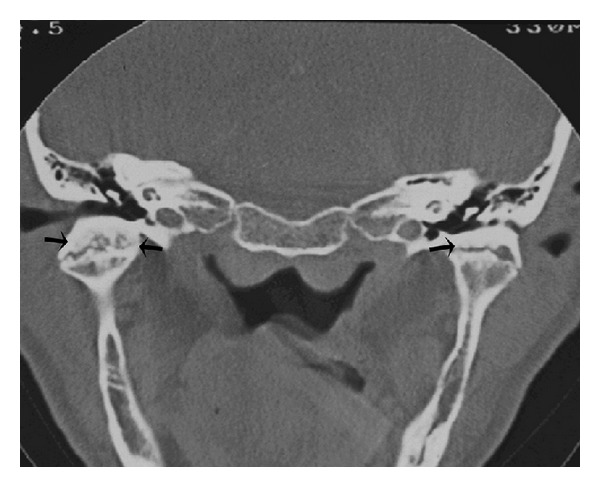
Coronal CT image demonstrating bilateral joint space narrowing, rough condylar surfaces, and sclerosis of the subchondral bone (arrows).
